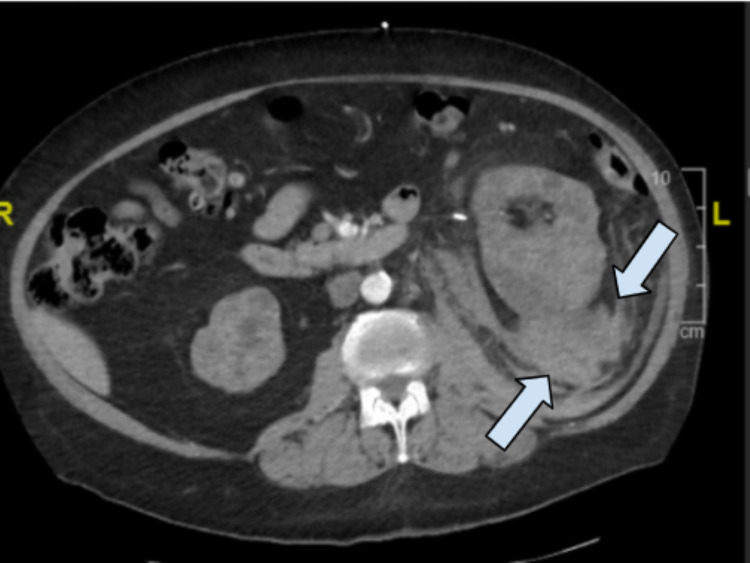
CT transverse imaging seven months prior to presentation Arrows show the 5.8 x 3.9 x 8.7 cm left perinephric hematoma
